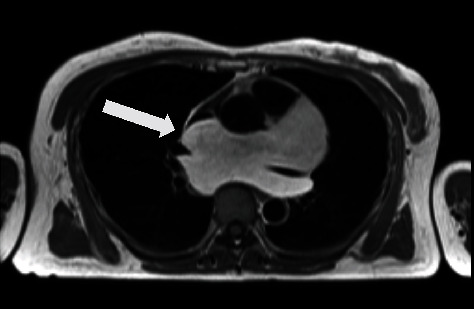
Preoperative magnetic resonance imaging findings. Arrows indicate mass.
